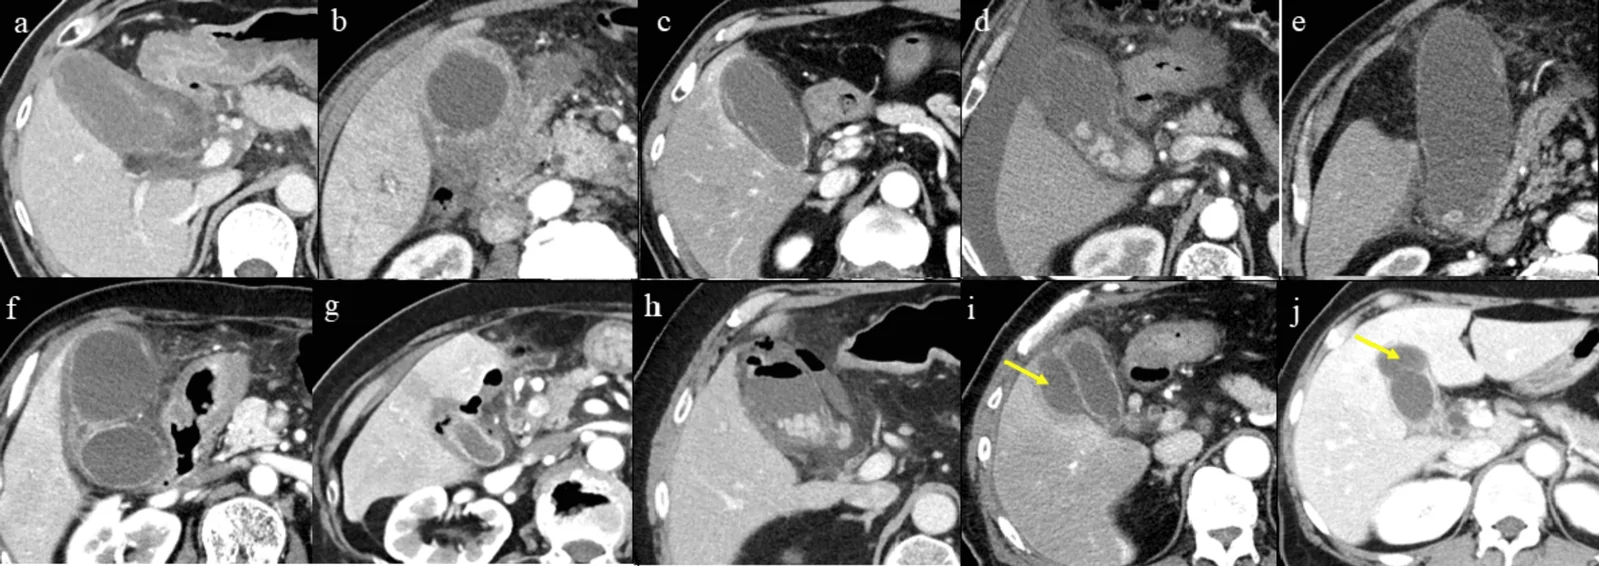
Representative preoperative CT findings used to assess local inflammatory severity in acute cholecystitis (a,b) Gallbladder wall thickening ≥5 mm. The thickened gallbladder wall is shown in two representative CT images. (c,d) Poor or absent gallbladder wall enhancement on contrast-enhanced CT, suggesting ischemic or gangrenous change. (e,f) Pericholecystic fat stranding extending ≥5 mm from the gallbladder wall, indicating surrounding inflammatory change. (g,h) Intraluminal or intramural gas within the gallbladder. (i,j) Pericholecystic abscess formation. Arrows indicate the abscess cavity CT, computed tomography
